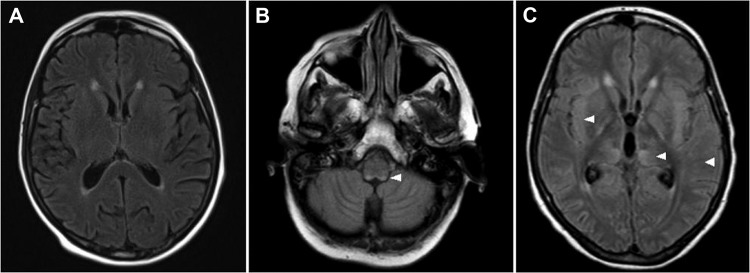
Magnetic resonance imaging axial flair sequence of brain of 66-year-old woman with fatal encephalitis, Bordeaux, France, 2012. A) No hypersignal at day 6. B) Bilateral posterior hypersignals in the medulla at day 13. C) Bilateral supratentorial hypersignals at day 24 in the cortex, the white matter, and the basal ganglia. Hypersignals are indicated by white arrowheads.
